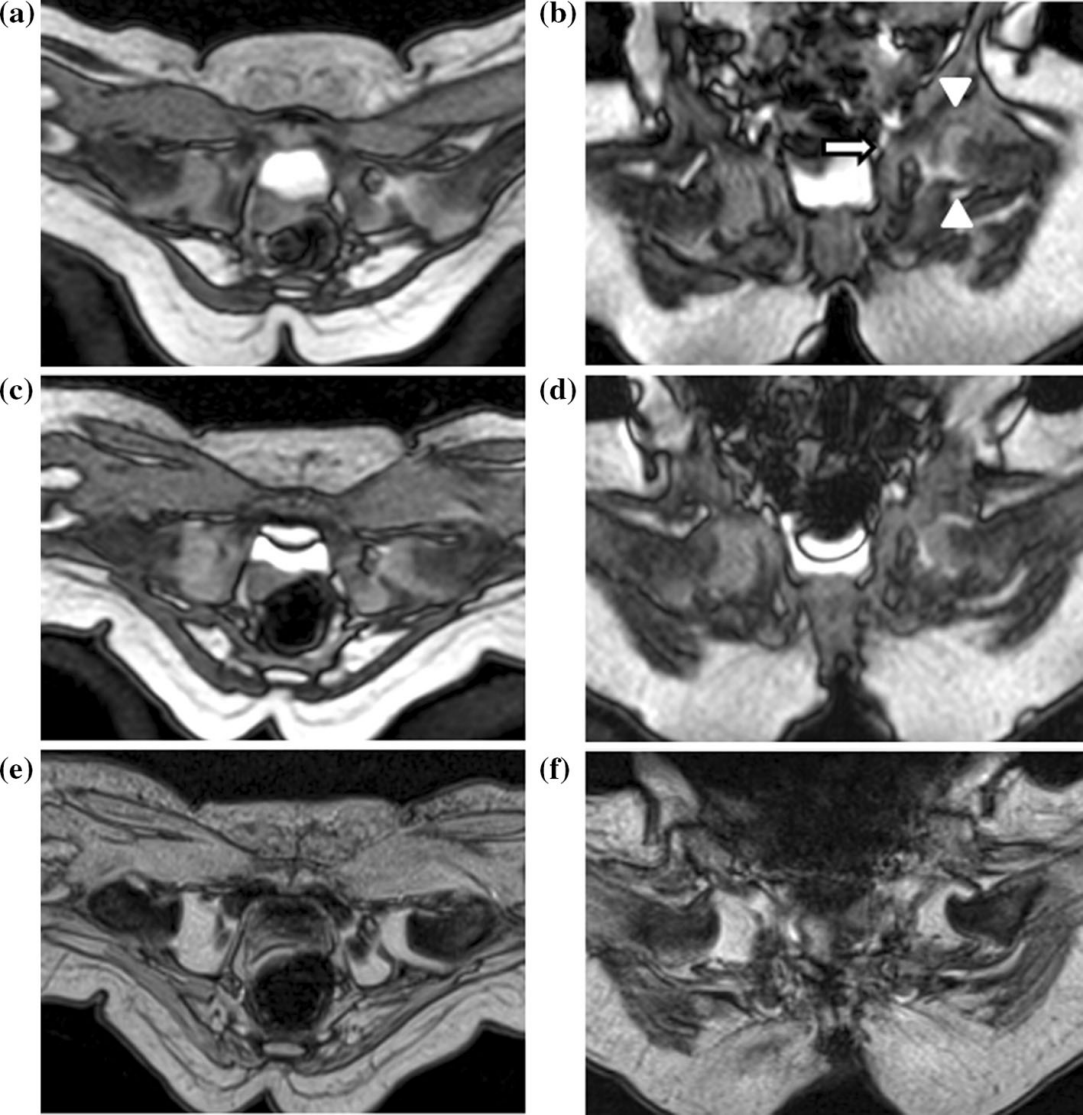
a, b Transverse and coronal images of the first ultrafast MRI study revealed an incomplete reduction of the left hip in a 6-month-old girl after gradual reduction with spica casting.* White arrow* and* arrowheads* Left triradiate cartilage and incomplete reduction of the left hip, respectively. The re-casting procedure was performed immediately after imaging. c–f Transverse and coronal images of the second ultrafast (c, d) and conventional MRI (MEDIC) (e, f) show successful reduction of the left developmental dysplasia of the hip. No motion artefact was observed on the ultrafast MRI
